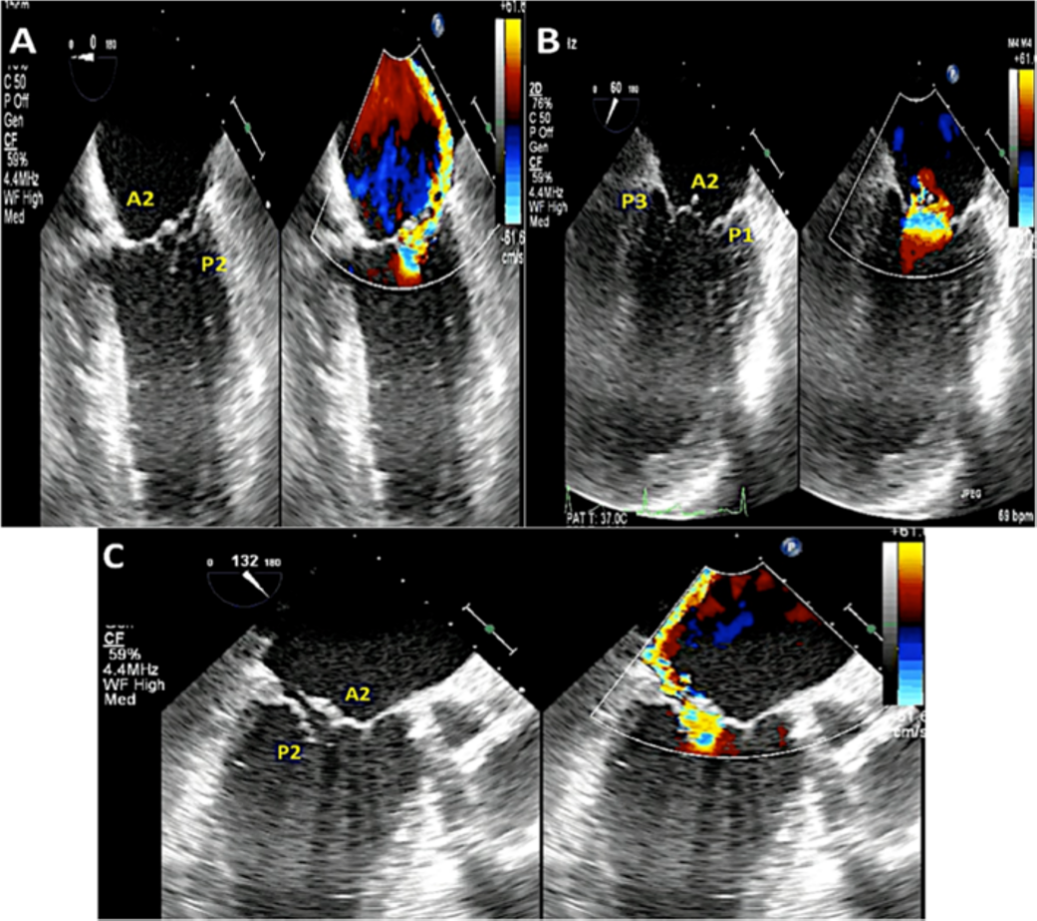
Multiplane 2D TEE with color Doppler is utilized to identify the mitral valve scallops. Panel A demonstrates a TEE at midesophageal 0 degrees view. In this view one can visualize the A2 and P2 scallops of the mitral valve at the tips of the leaflets. However the scallops visualized depend on the level of depth. At 0 degrees midesophageal view, when the aortic valve is visualized, the A1/P1 scallops are noted at the leaflet tips. When the TEE probe is advanced further into the esophagus past the midesophageal level, the A3/P3 scallops are identified at the leaflet tips [16]. Of note, the A2 scallop of the mitral valve is flail with a posteriorly directed mitral regurgitation jet. Panel B demonstrates a commissural view at 60 degrees. In this view, the lateral most scallop (close to the appendage) is P1. One can also visualize the central, flail A2 scallop. The P3 scallop is also well visualized in this view. The mitral regurgitation originated around the A2 scallops as was seen in the 0 degree view. Panel C is the long axis view between demonstrating the A2 and P2 scallops.
